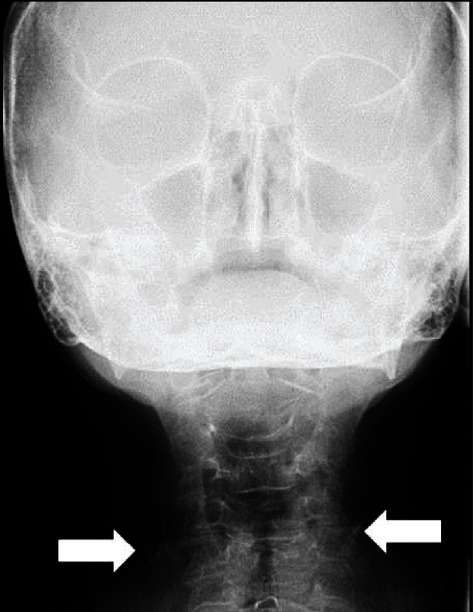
Manzi Projection showing the presence of bilateral radiopaque masses, adjacent to the cervical region (arrows). Notice that these structures are inferior to the calcified structure region demonstrated by the panoramic radiograph.
